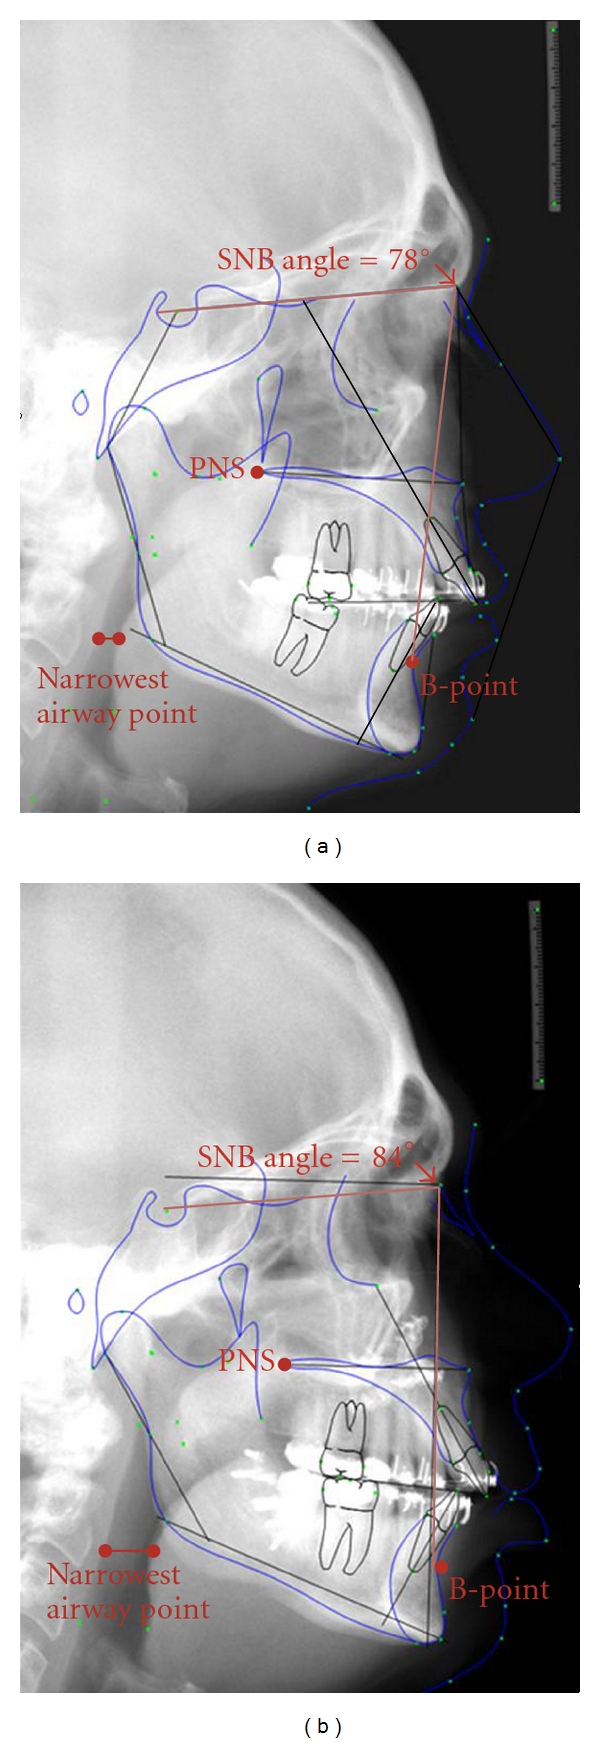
Pre- (a) and post- (b) maxillomandibular advancement (MMA) lateral cephalometric radiographs demonstrating analyzed parameters. Pre- and post-MMA cephalometric images were digitally overlaid to determine the change in position of the following parameters: anatomic B-point (most posterior midline point in the anterior concavity of the mandible); posterior nasal spine (PNS) along the sella-nasion line; and sella-nasion-point B (SNB) angle. Enlargement of the posterior airway space was also determined on all cephalometric radiographs by measuring the narrowest point in the airway pre- and post-MMA, then subtracting the larger (post-MMA) value from the smaller (pre-MMA) value to obtain the difference.
